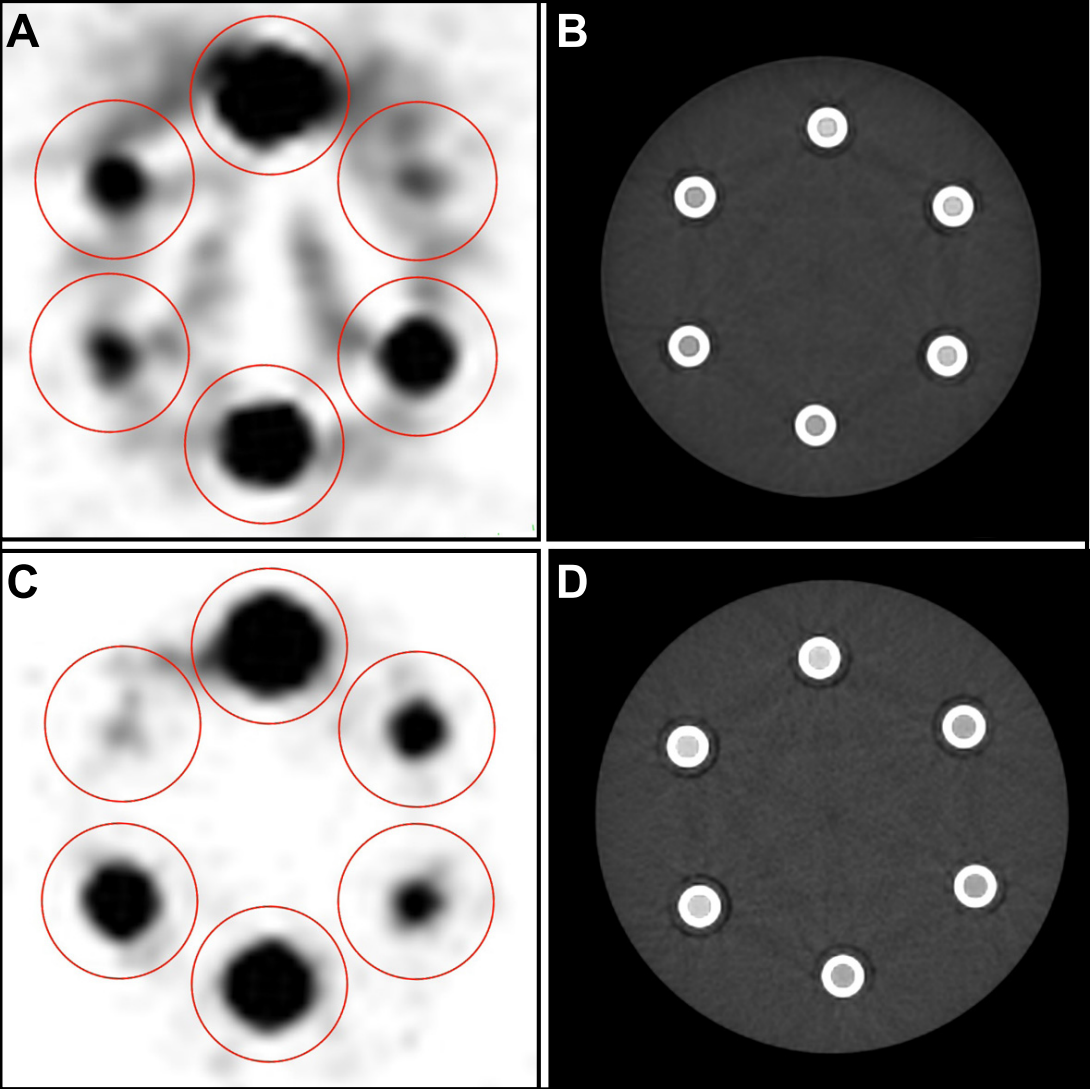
Examples of PET and CT images of axial slices acquired from a scanner of vendor A (a, b; obtained from scanner number 5) and vendor B (c, d; obtained from scanner number 15) to emphasize the differences between the two scanner vendors. Both scanners were calibrated shortly after each other, so decay of the reference activities was negligible. At the time of calibration, the top vials contained a reference activity *A*
